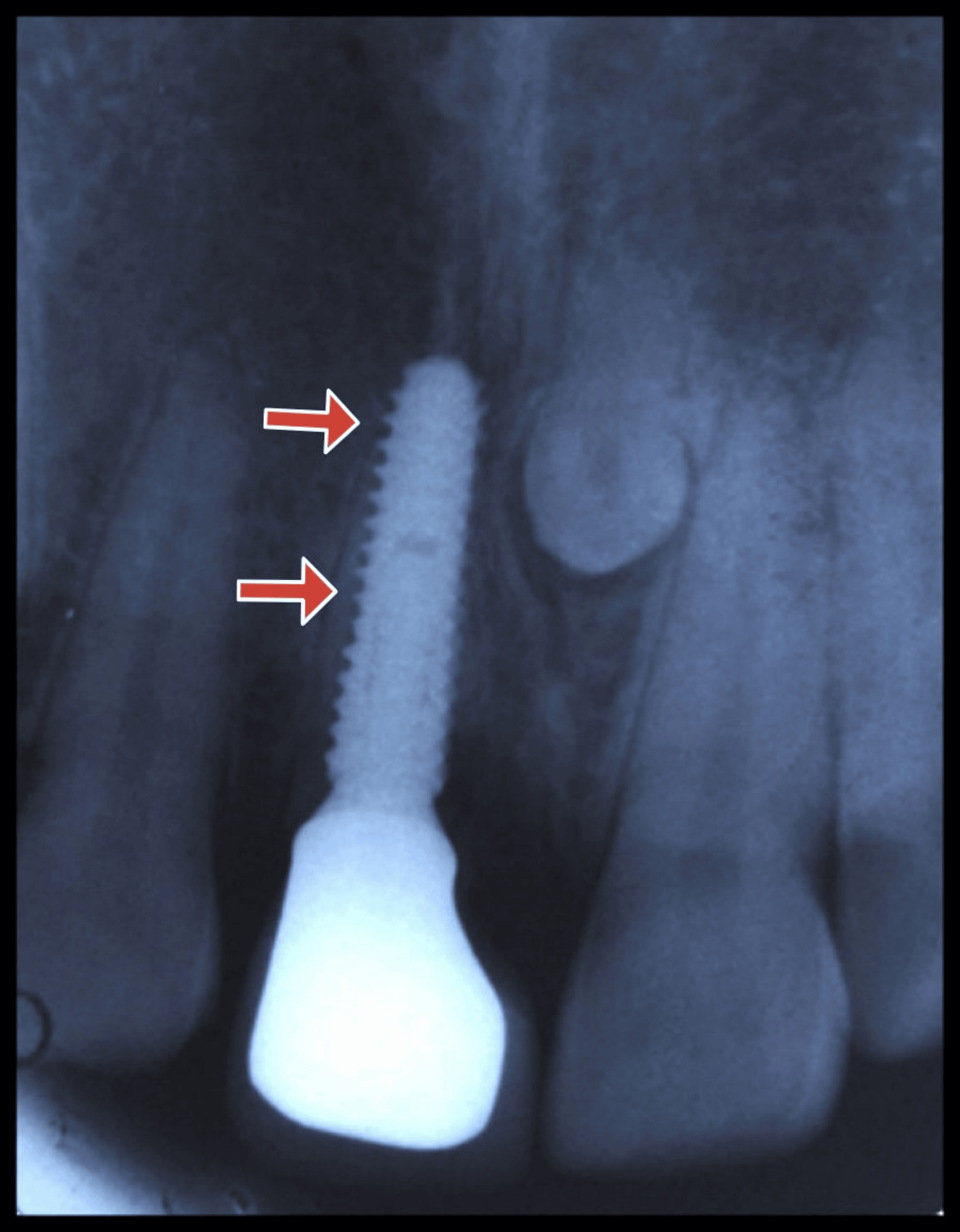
Case 2: During the follow-up visit one year after loading, IOPA was taken, which shows the intact buccal shield and a negligible amount of bone loss in relation to 11 IOPA: Intra-oral periapical radiograph.
